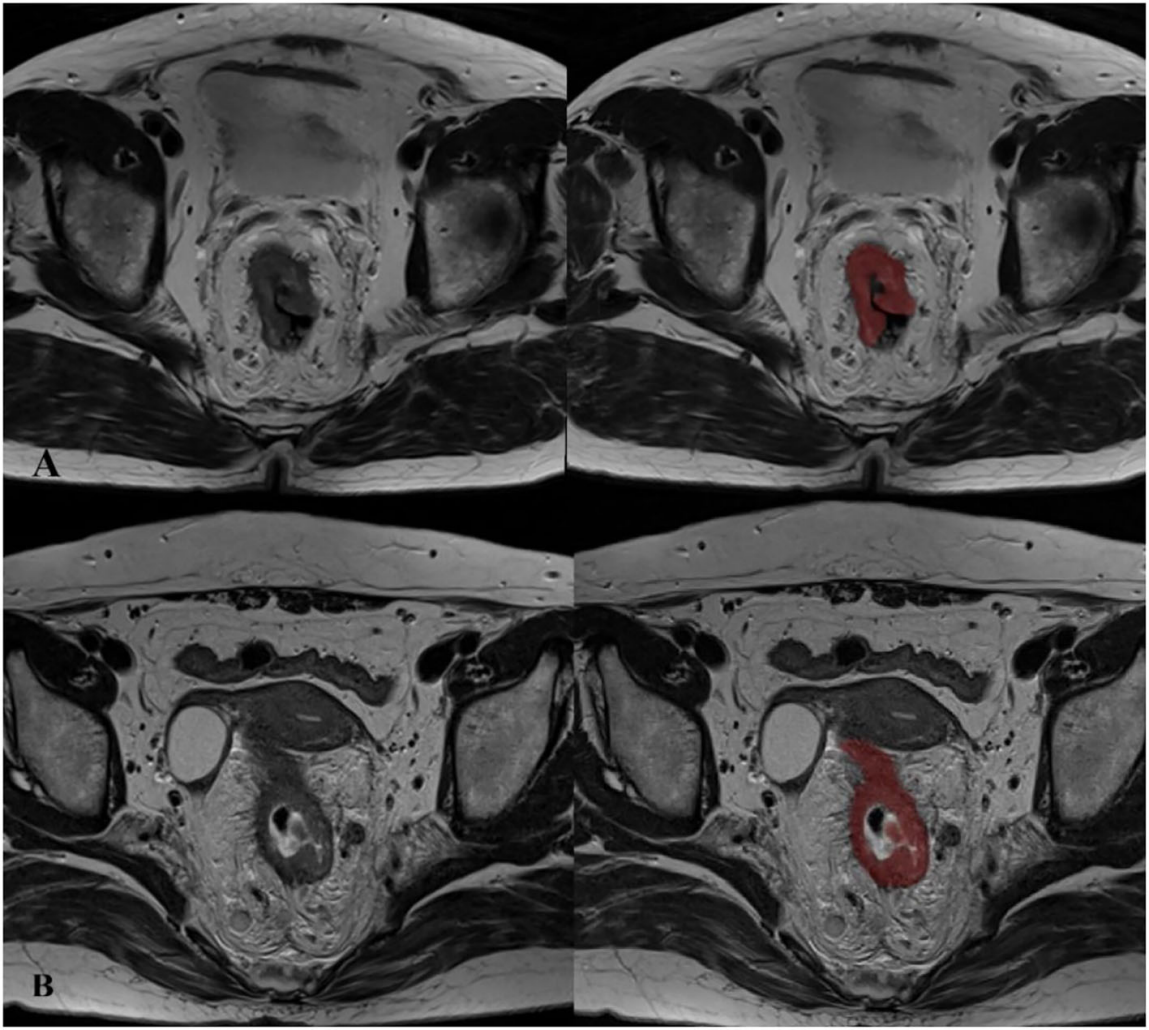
Examples for gross tumor volumetry in rectal cancer on axial contiguous MR images. (A) A 69-year-old man with a tumor in the mid-rectum, before treatment staged as T3N2, EMVI (+), CRM (+), TD (-). (B) A 57-year-old woman with a tumor in the low-rectum, before treatment staged as T4N2, EMVI (+), CRM (+), TD (+).
